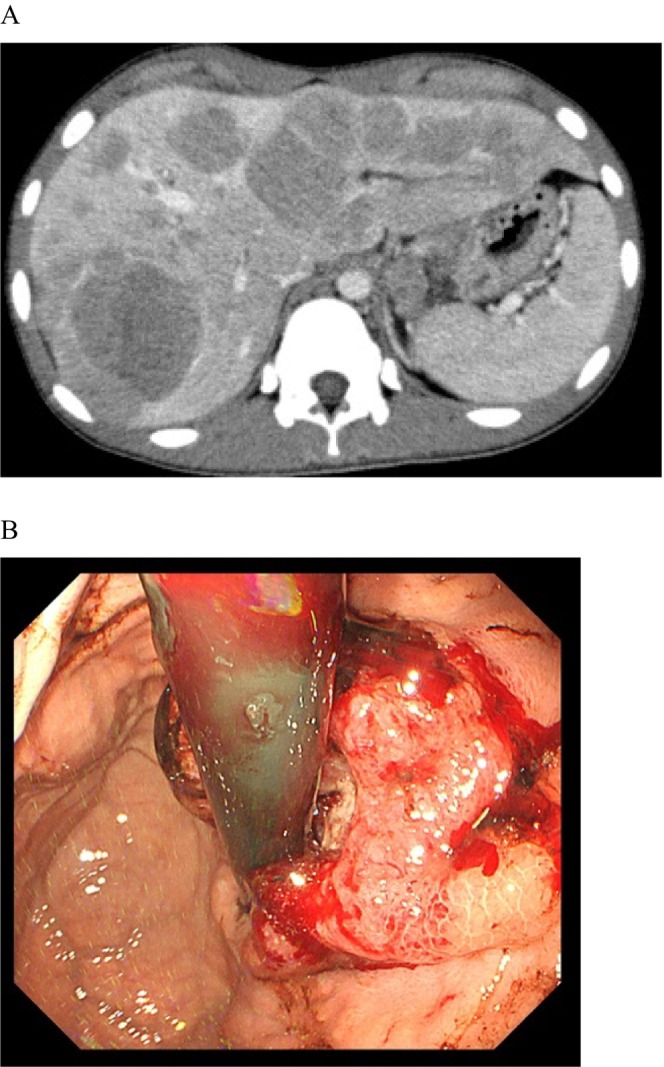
(A) CT tomography revealed mucosal thickening around the gastric cardia and enlarged lymph nodes on the lesser curvature. Multiple occupying lesions with ring enhancement were observed in both the lobes of the liver. (B) Gastrointestinal endoscopy revealed a 3/4 circumferential ulcerative lesion with a surrounding wall on the lesser curvature of the cardia. A tumor biopsy revealed moderately differentiated tubular adenocarcinoma and poorly differentiated adenocarcinoma.
